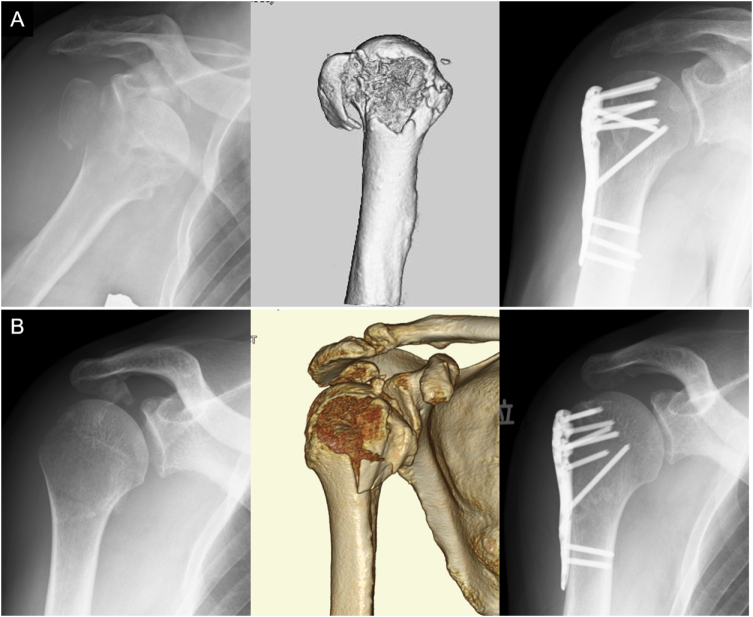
Radiographs taken before and after surgery. (A) 72-year-old man presented with right proximal humeral fracture-dislocation with split fracture of greater tuberosity (left panel). After reduction, computed tomography revealed a proximal humeral fracture with a displaced greater tuberosity fragment and minimal head-shaft displacement (middle panel). Postoperative radiograph of osteosynthesis using a plate and suture bridge technique (right panel). (B) 70-year-old man presented to our hospital after undergoing closed reduction for proximal humeral fracture-dislocation at the other hospital (left panel). Computed tomography showed a proximal fracture-dislocation with an avulsion fracture of greater tuberosity (middle panel). Postoperative radiograph after osteosynthesis using a plate and suture bridge technique (right panel).
